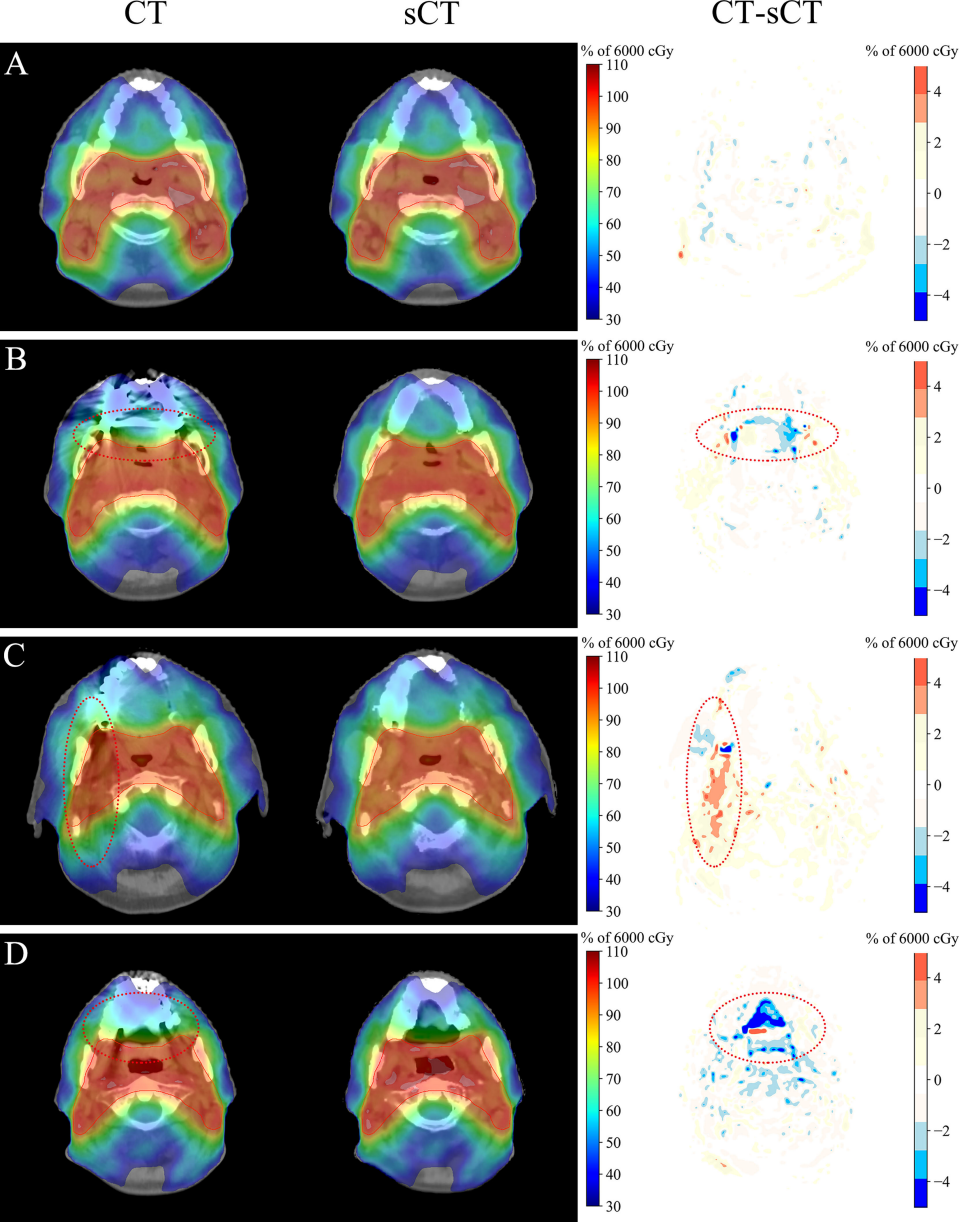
Dosimetric comparison of CT and sCT images. (A) Metal-free images. (B–D) Metal-containing images. The display window for the CT and sCT images was [-360, 440] HU. The dose distribution of sCT_MF images was consistent with that of CT_MF images, and there were obvious dose differences between CT_M and sCT_M images in the area with serious metal artifacts.
